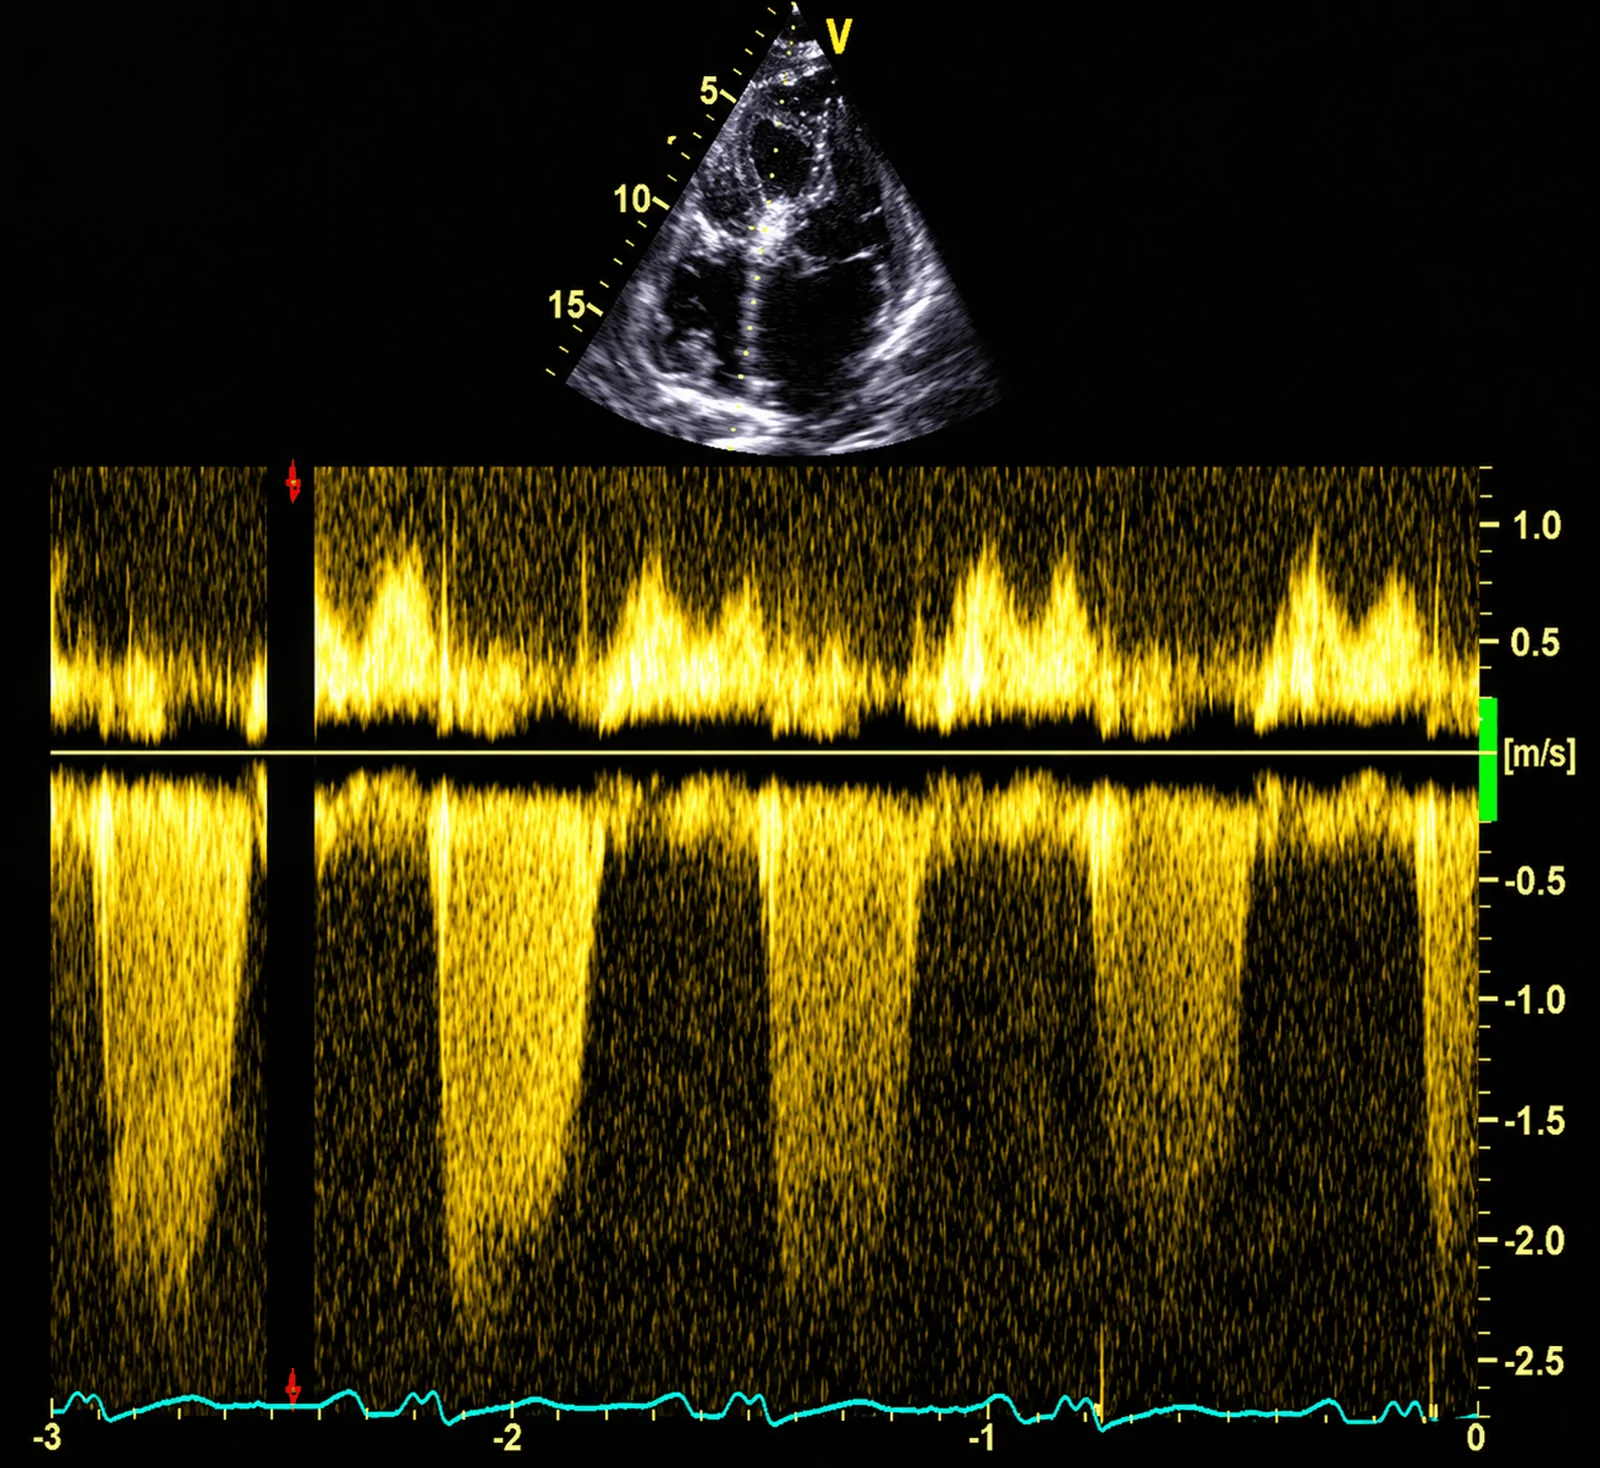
Doppler assessment of tricuspid regurgitation Continuous-wave Doppler transthoracic echocardiography showing moderate tricuspid regurgitation secondary to interaction between the migrated venous stent and the tricuspid valve apparatus. The maximal tricuspid regurgitation velocity was estimated at 2.7 m/s, with an estimated systolic pulmonary artery pressure of approximately 30 mmHg.
